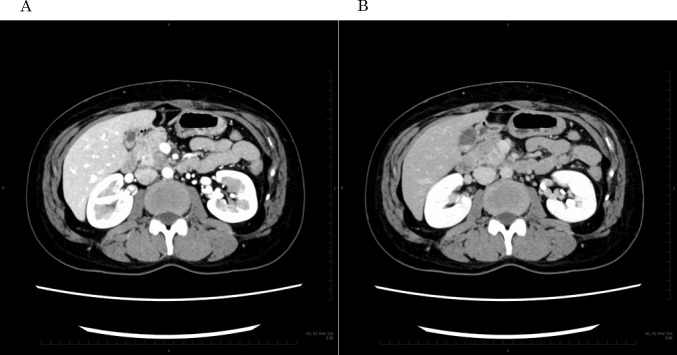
Contrast enhanced CT. A: early phase, B: late phase. Mass is gradually enhanced (circle)
